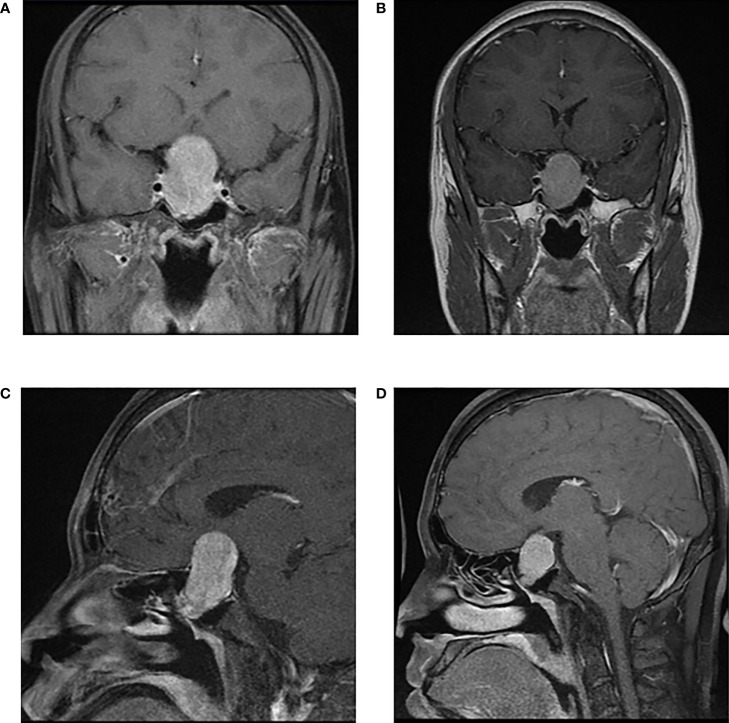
A patient with macroadenoma (46.7×27.7×23.5mm) received long-term SSA (Sandostatin LAR) for 12 weeks and achieved tumor shrinkage (34.2×24.8×20.9mm). Thyroid functions reduced to normal ranges after 4 weeks of medication. However, tumor was in tough texture examined in surgery and rather hard to resect due to long duration of SSA use. | Enhanced Pituitary MRI before SSA: (A) axial view and (C) sagittal view; Enhanced Pituitary MRI after SSA: (B) axial view and (D) sagittal view.
